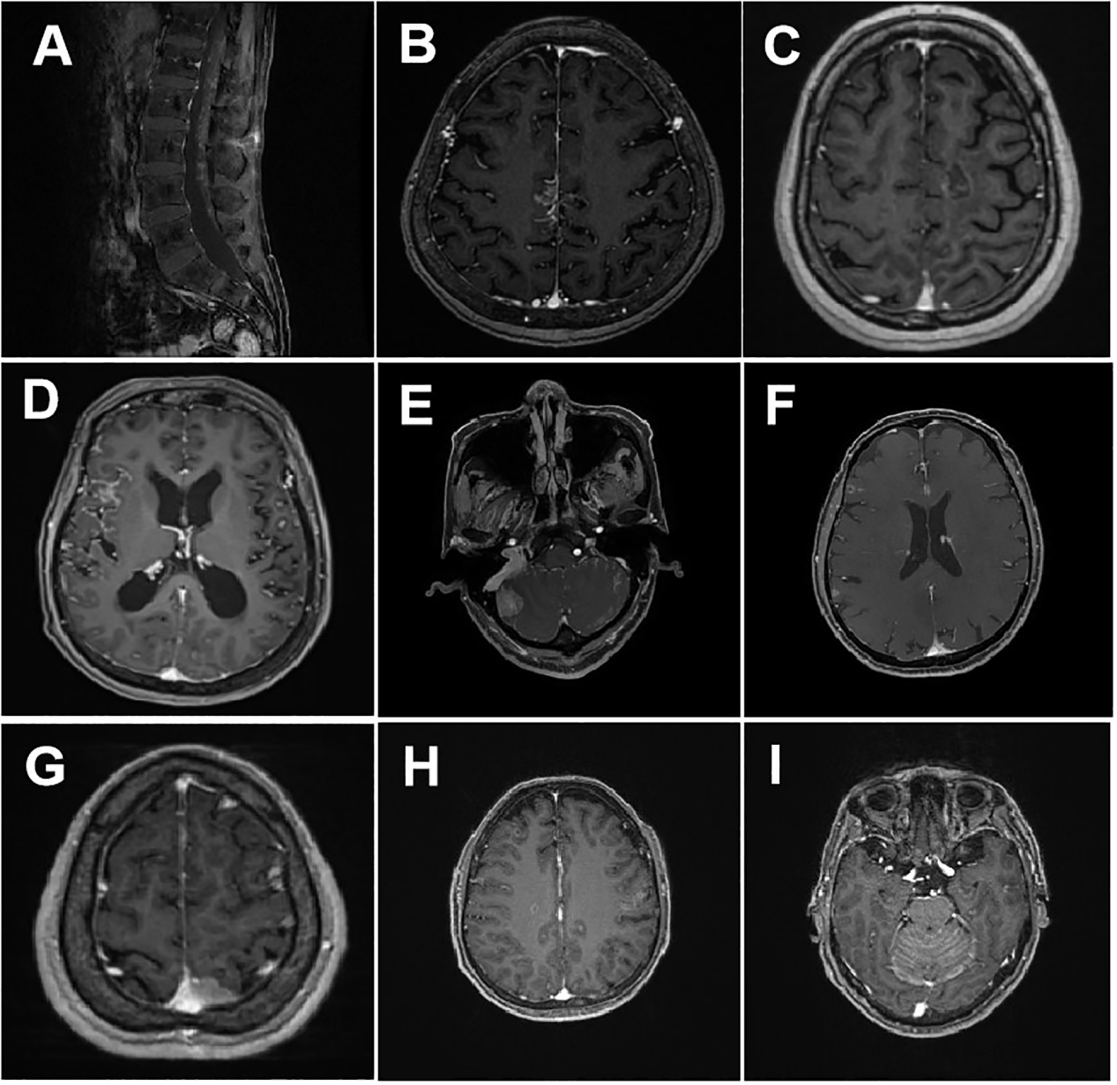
Representative brain MRI of patients with leptomeningeal metastases. (A) Meningeal metastasis spreading to the spinal cord, with extensive enhancement of the spinal cones and multiple tubercles in the cauda equina; (B) Enhanced MRI showing thickening of the meninges in the right upper frontal midline region, with a slightly decreased T1W signal, a slightly increased T2W signal, and a high T2 flair signal; (C) Cranial parenchyma enhancement displaying mild ring-shaped enhancement, with a large patchy high-signal edema band on T2FLAIR surrounding the nodule; (D) The meninges of the right frontotemporal lobe showing linear enhancement, along with enlargement of the ventricular system; (E) The right cerebellar hemisphere showing a patchy low-signal on T1WI, high-signal on T2WI, low signal on DWI, high signal on T2 FLAIR, and marked enhancement on post-contrast imaging; (F) The brain parenchyma and cerebral hemispheric meninges showing uneven thickening and marked enhancement; (G) The left parietal dura exhibiting nodular thickening with prominent enhancement; (H) Multiple abnormally enhanced nodules were observed in both hemispheres; (I) The cerebellar hemispheres displaying multiple abnormally enhanced nodules, with diffuse thickening and enhancement of the cerebellar meninges.
